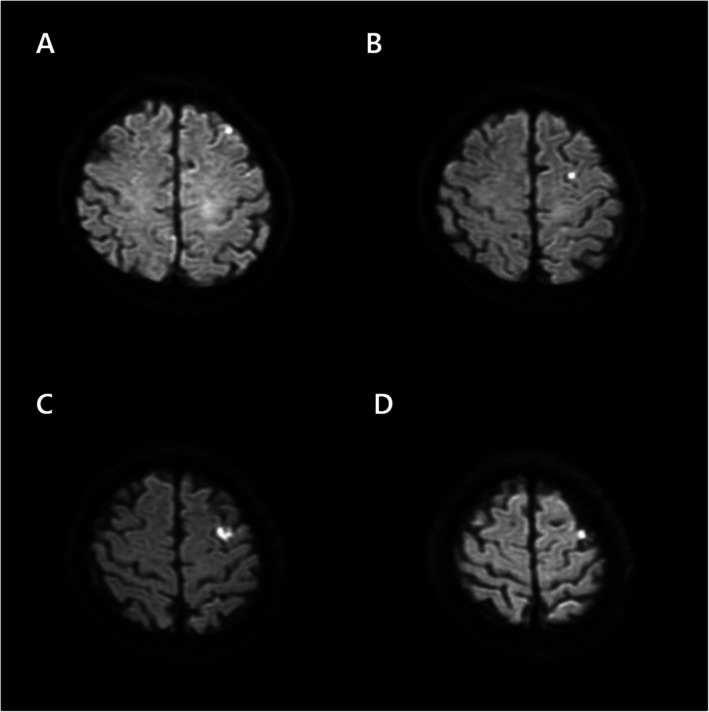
Diffusion‐weighted magnetic resonance imaging demonstrating acute cerebral infarction. (A–D) Diffusion‐weighted images showing high‐signal‐intensity lesions in the cortical–subcortical watershed zone between the anterior cerebral artery and middle cerebral artery territories, involving the superior frontal and parietal lobules.
